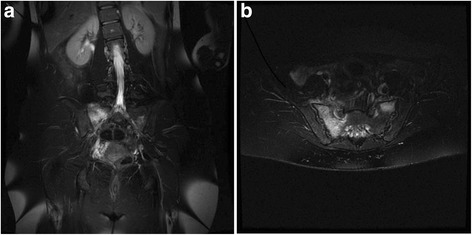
MRI of pelvis. a coronal T2 demonstrating bone marrow edema with non-displaced fracture line on right sacrum. b axial T2 demonstrating bone marrow edema in both sacral bones
